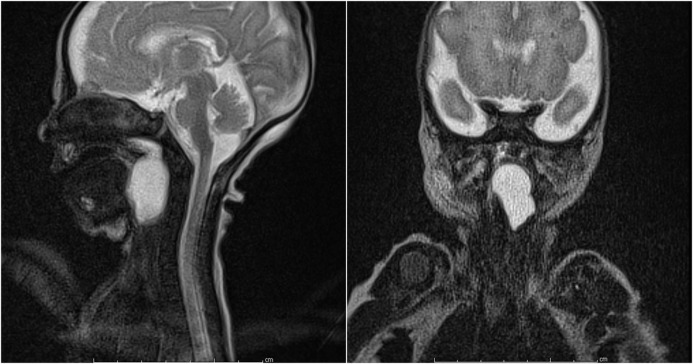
Figure 1: Magnetic resonance images demonstrating the vallecular cyst.
